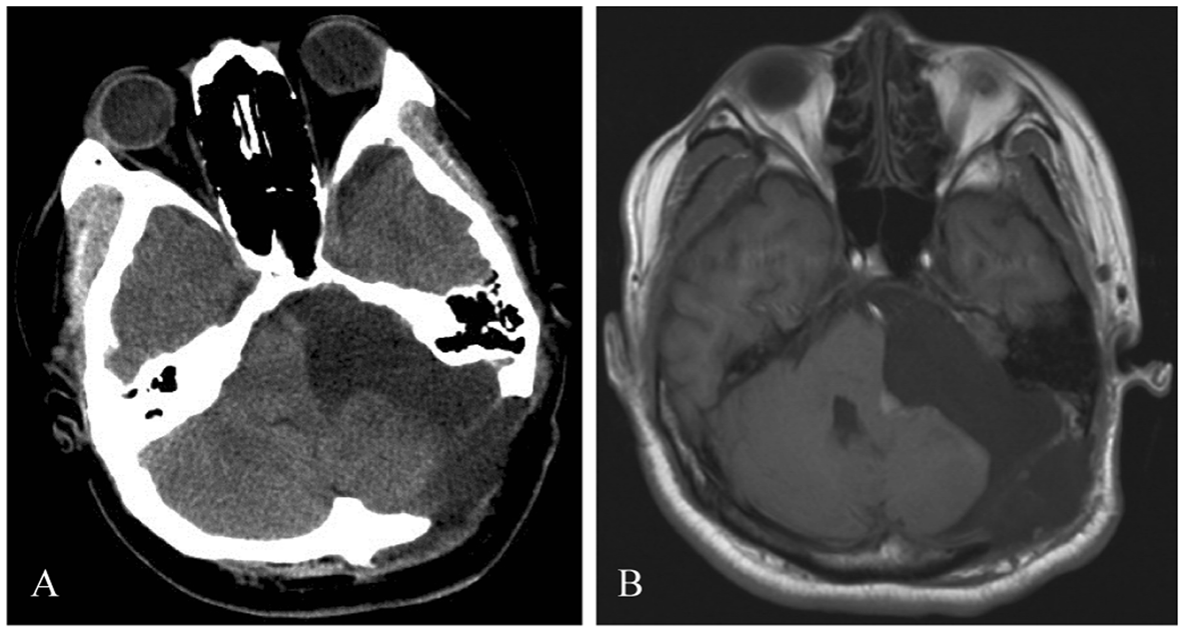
Postoperative CT (A) and MRI (B) showing complete removal of the tumor.
